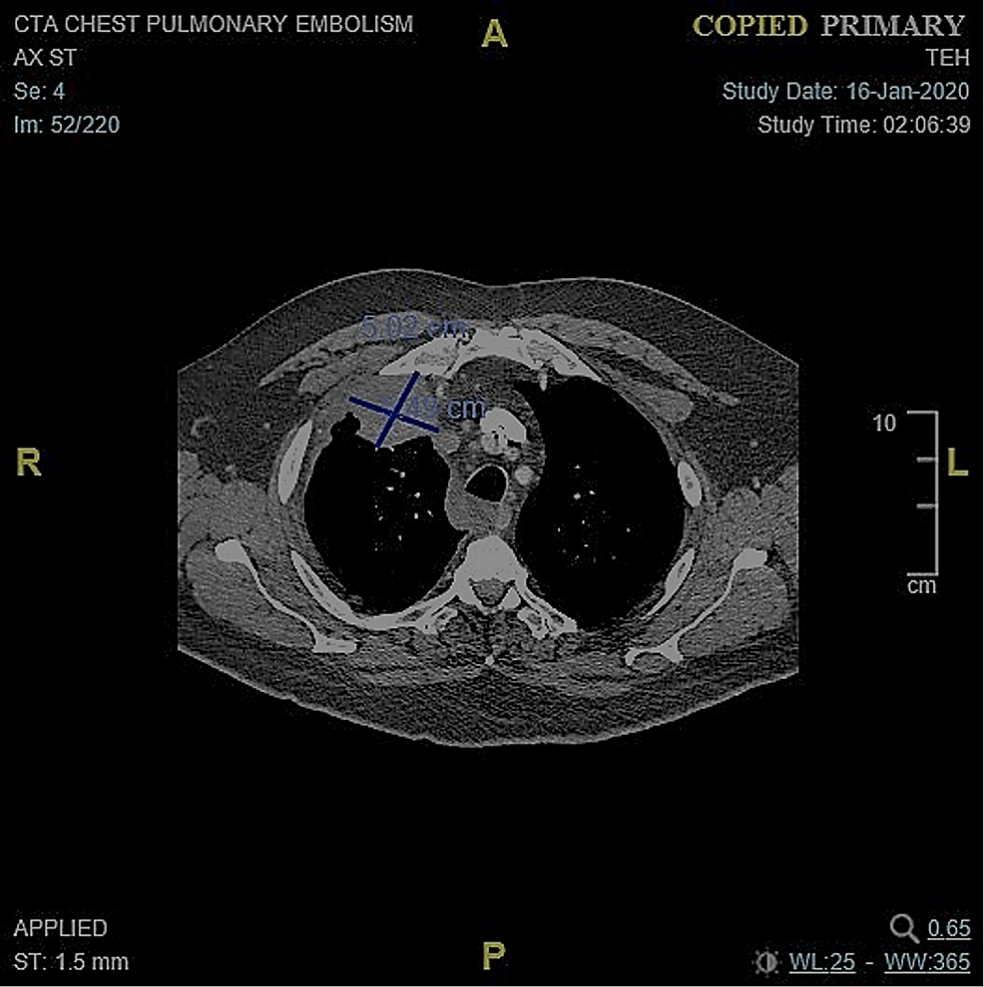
Initial CT chest with tumor measuring 5.02 cm x 5.49 cm
